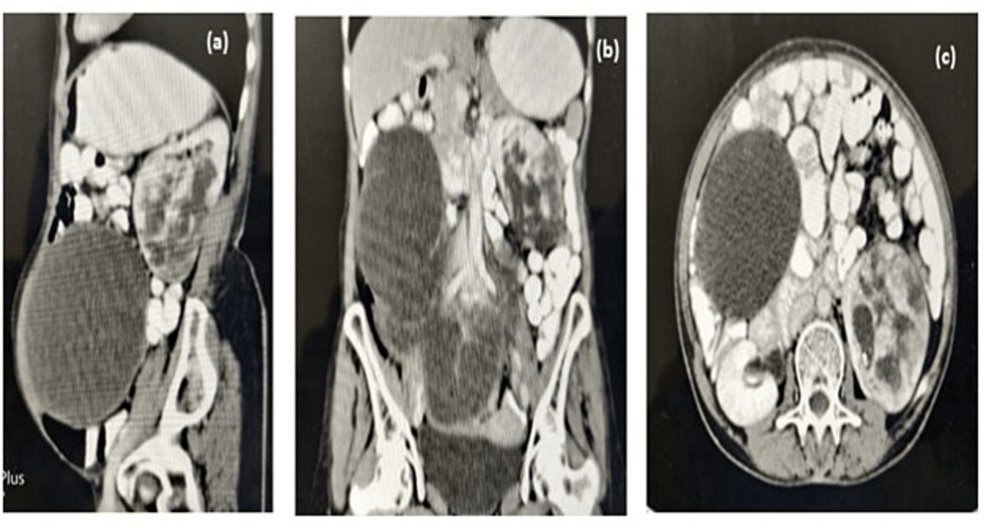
CECT images (a, sagittal plane; b, coronal plane; c, axial plane) showing 13 x 11 cm mass of the left kidney. CECT: contrast-enhanced computed tomography
